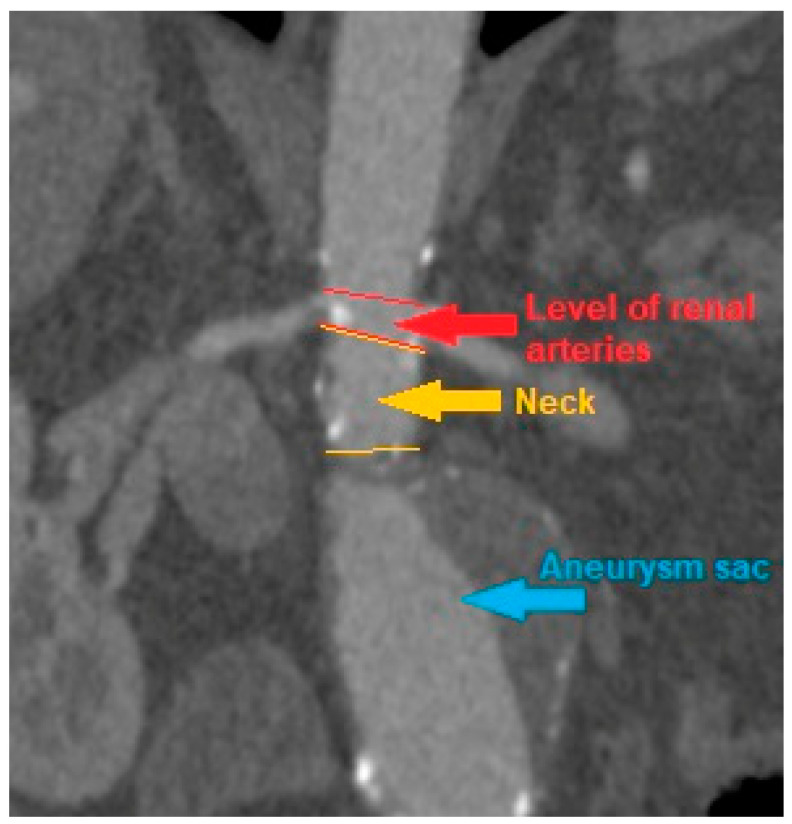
An example of computed tomography of patient with infrarenal abdominal aortic aneurysm with present neck segment.
